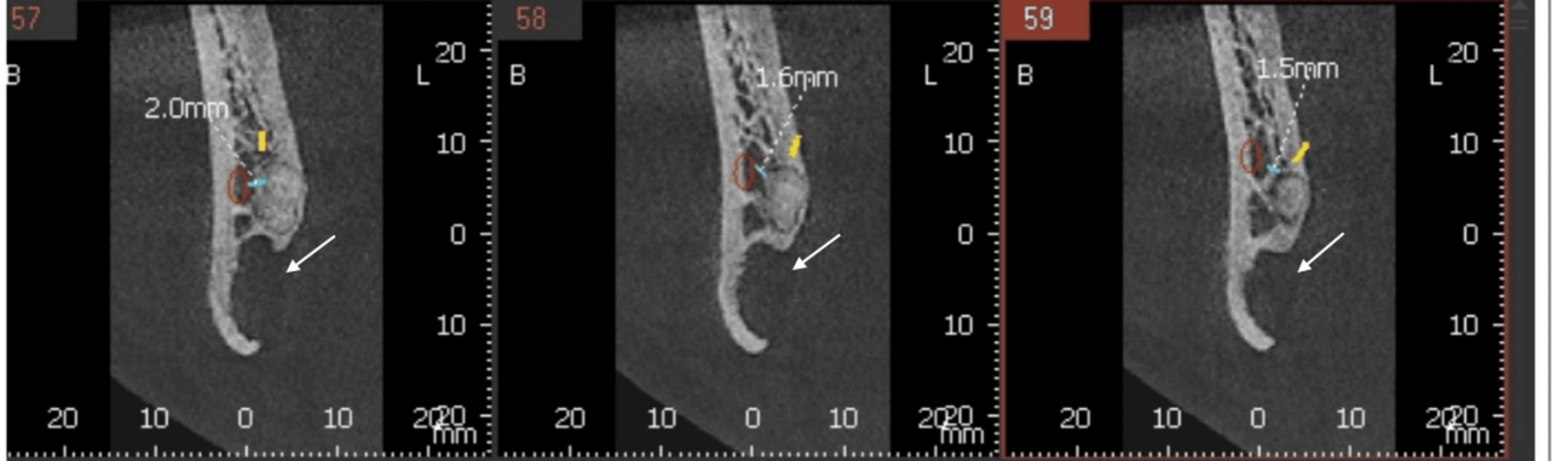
Cone-beam computed tomography of the patient (white arrows show the Stafne cyst)
